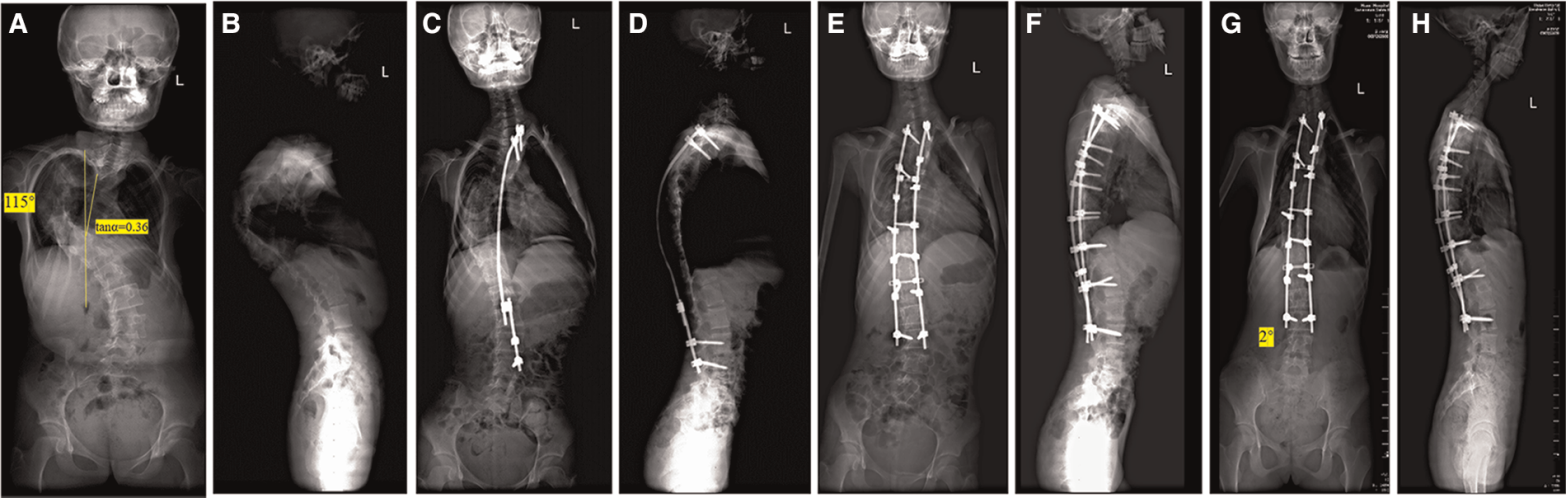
A 16-year-old girl with a severe and rigid thoracic curve (A,B). The radiographs demonstrate a thoracic curve angle of 115°, and the slope of the line connecting the pedicles on the concave side of the upper- and lower-end vertebrae (Tan *α*) is 0.36. After-distraction posteroanterior (C) and lateral (D) radiographs demonstrate well-maintained global coronal and sagittal balance. Postoperative posteroanterior (E) and lateral (F) radiographs following posterior spinal fusion from T2 to L3 demonstrate well-maintained global coronal and sagittal balance. Radiographs made at the final follow-up (G,H) reveal well-maintained global coronal and sagittal balance with absence of the distal adding-on phenomenon.
